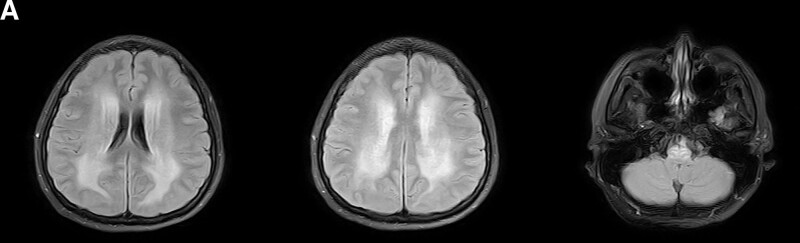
T2 fluid-attenuated inversion recovery magnetic resonance imaging (MRI) on admission shows diffuse bilateral, symmetric hyperintense signal abnormality involving both white matter, medulla oblongata, and brainstem (A).
